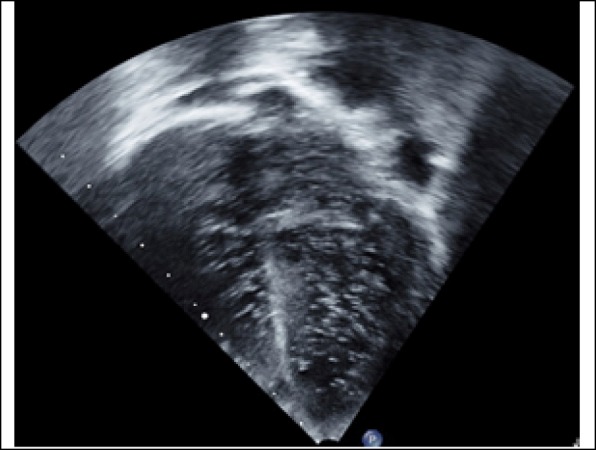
Trans-thoracic echocardiogram in four-chamber view with bubbles after injection of agitated saline. Opacification of the right atrium and right ventricle was noticed immediately. It is followed within one to two beats by complete opacification of the left atrium and left ventricle, indicating significant intrapulmonary shunting
